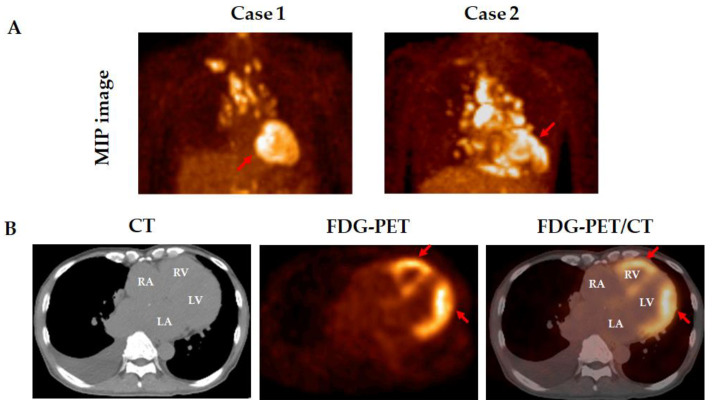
Representative sarcoidosis cases with FDG uptake in the heart. 18F-Fluorodeoxyglucose-positron emission tomography (FDG-PET) showing FDG uptake in the left ventricle (A). FDG uptake is shown in the right and left ventricle (B). FDG uptake lesion were pointed by red arrow. CT, computed tomography; MIP, maximum intensity projection; RA, right atrium; RV, right ventricle; LA, left atrium; LV, left ventricle.
